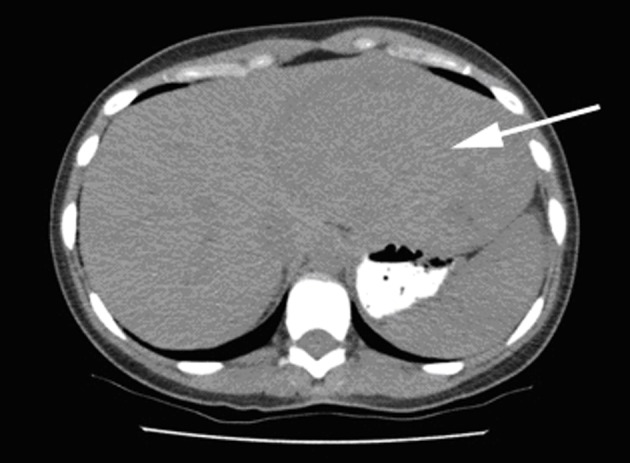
Liver lesion: CT image showing large hypodense lesion in the left liver lobe (arrow).
